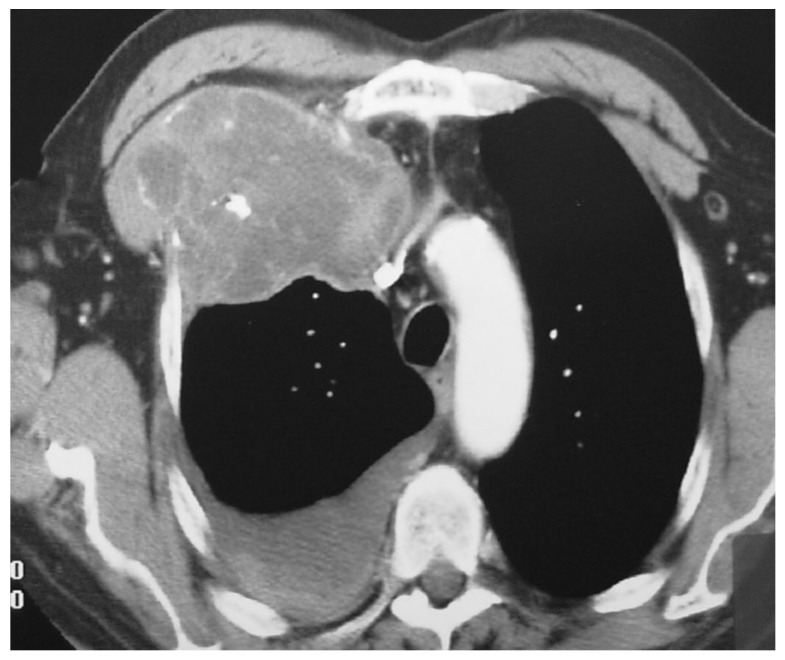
A 72-year-old male patient with grade 2 costochondral junction chondrosarcoma. Contrast-enhanced axial chest CT shows a large (90 mm) soft tissue mass at the right costochondral junction with small calcifications, necrotic areas, mediastinal invasion and right pleural effusion.
